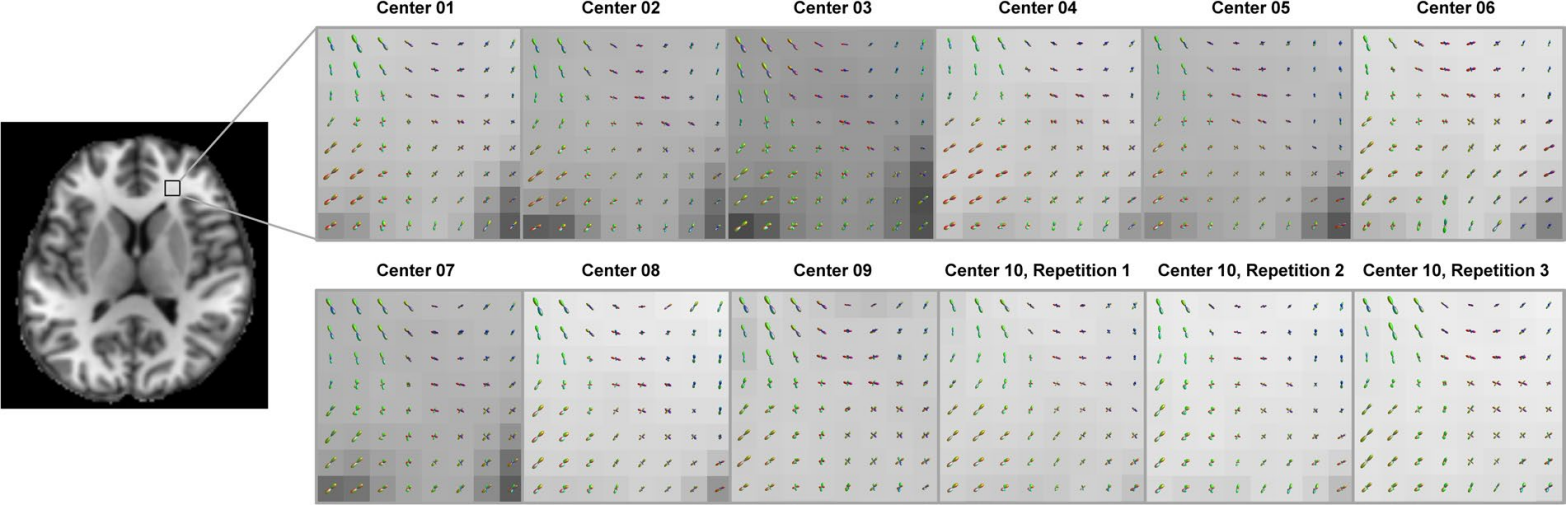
Fiber orientation distribution (FOD) from subject 1. The FODs are overlaid with registered T1-weighted images.Track density imaging (TDI):
